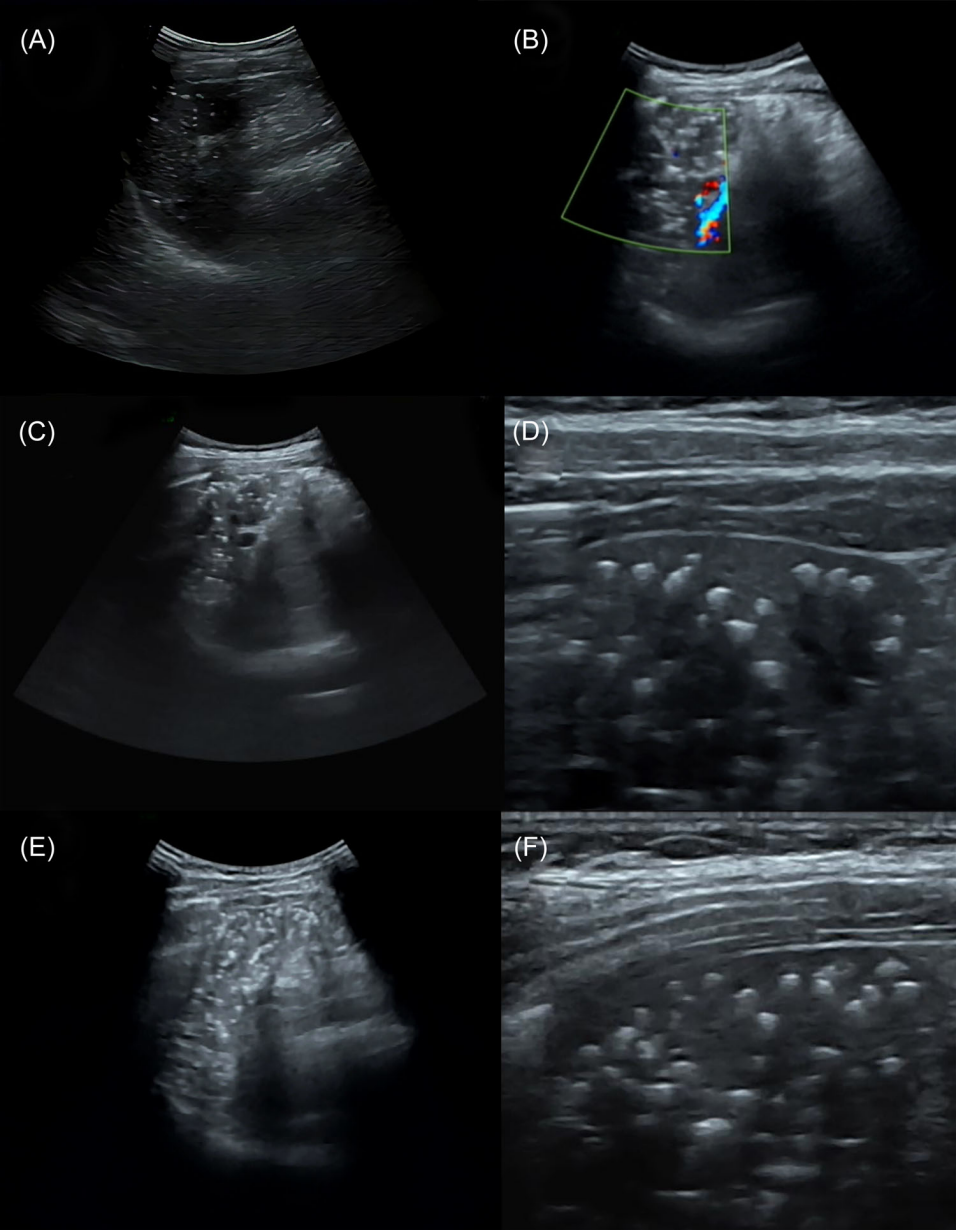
Ultrasound examination of the spleen from 2016 to 2023. (A) Ultrasound examination in 2016 indicated the parenchymal echo of spleen was uniform, and multiple punctiform strong echoes could be detected internally, with the maximum being about 3 mm, some of which were accompanied by the comet–tail sign; (B) Colorful ultrasound examination in 2020 showed that the number of punctiform strong echoes in the splenic parenchyma increased significantly compared with 2016, and the diameter increased slightly; (C, D): Ultrasound examination in 2021 demonstrated that the strong echoes in the splenic parenchyma have increased to the maximum diameter of 3.4 mm; (E, F): Ultrasound examination in 2023 indicated that the strong echoes in the splenic parenchyma increased significantly, with a maximum diameter of 3.7 mm.
